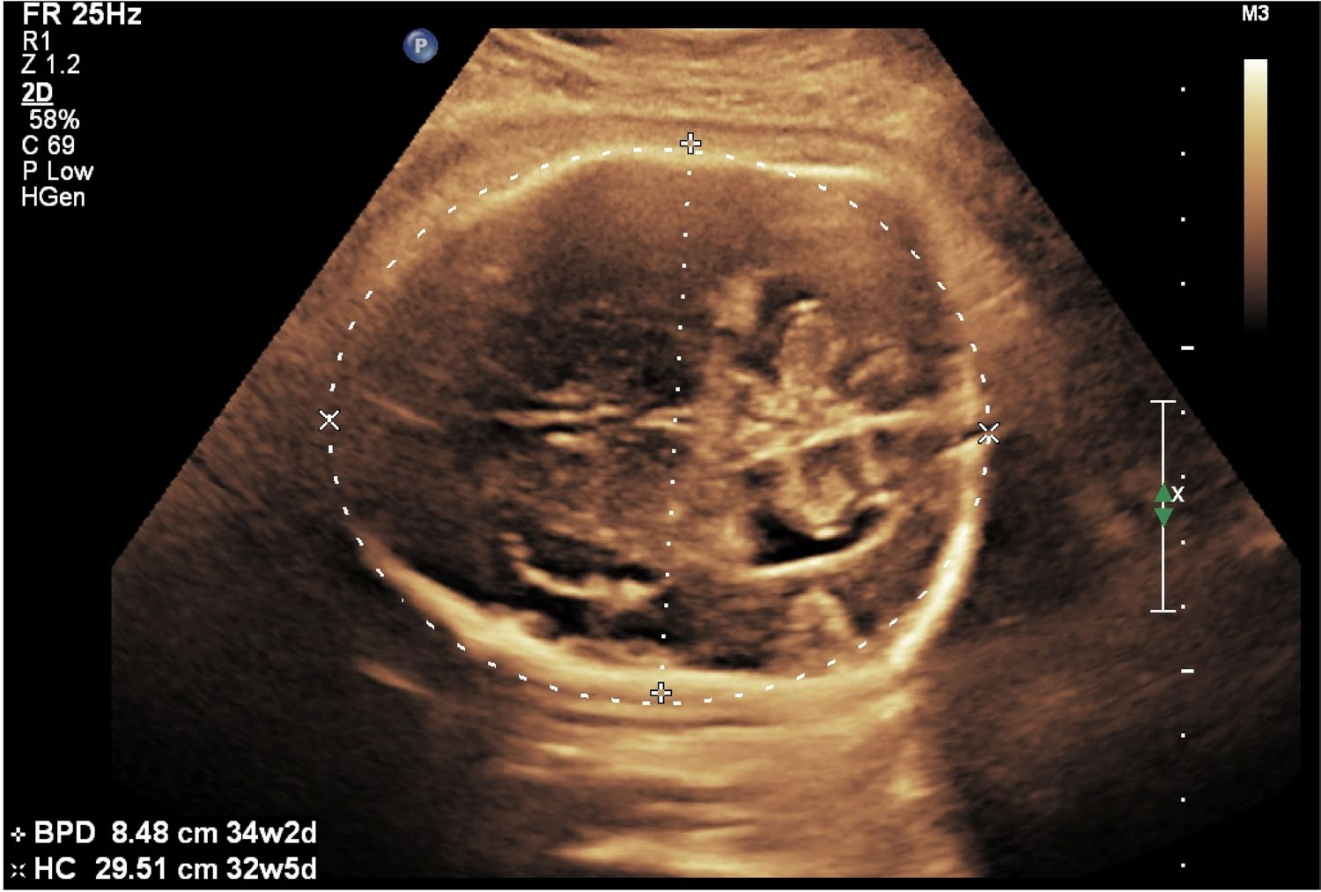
Fetus with bilateral coronal synostosis caused by Crouzon syndrome. The ultrasound shows a flattened occiput and mild bilateral frontal depressions of the skull
